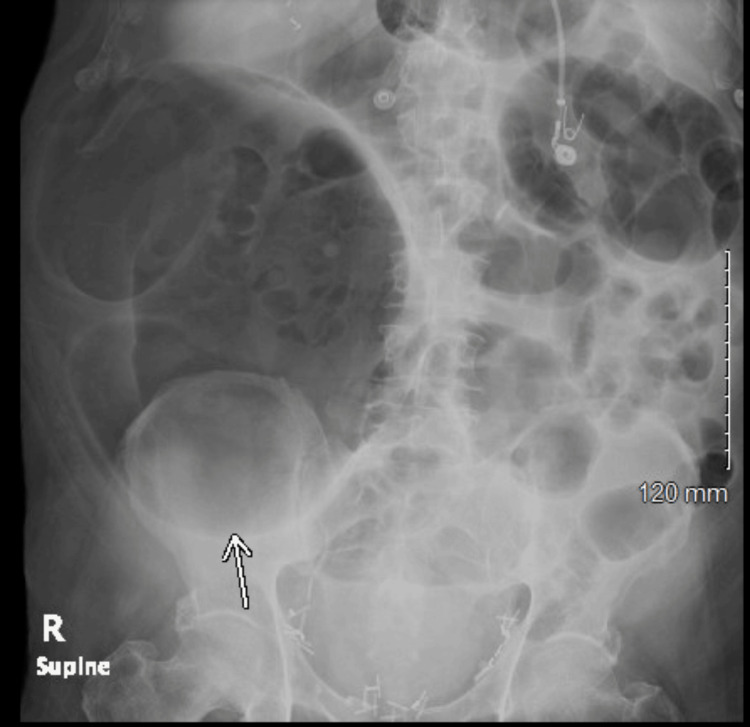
Abdominal X-ray showing dilation of the cecum (white arrow)
